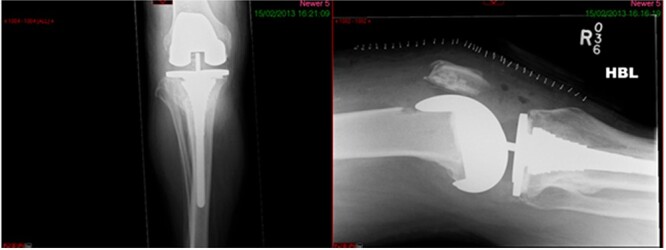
Immediate post-op radiograph.
